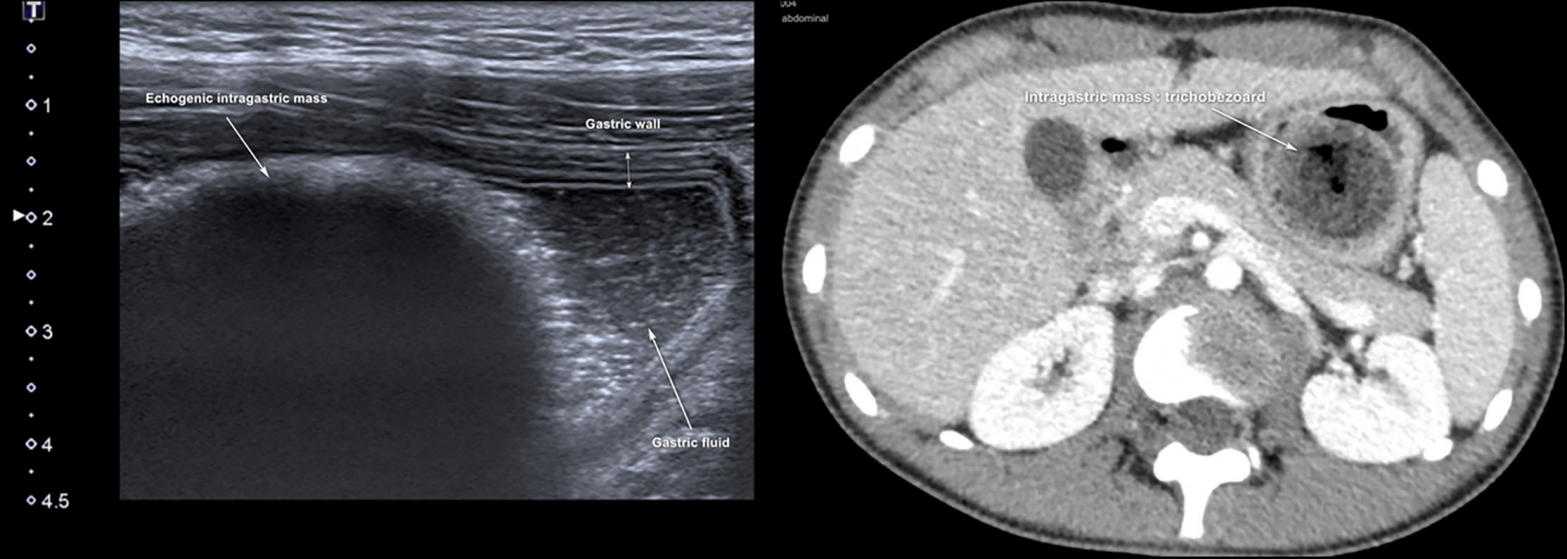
Intragastric foreign body. Large hyperechoic mass within the stomach may suggest the diagnosis of an intragastric foreign body (e.g., voluminous trichobezoar). In an uncertain diagnosis, and before surgery, an abdomino-pelvic CT scan should be performed to examine the extension of the foreign body. Gastric POCUS techniques found in the literature: various ultrasound probes have been used (high- or low-frequency linear or curvilinear transducers) and the child was positioned in a supine and/or a right lateral decubitus to enhance the quality scanning of the thoracic and epigastric areas. Liquid filling may help in visualizing the foreign body.
